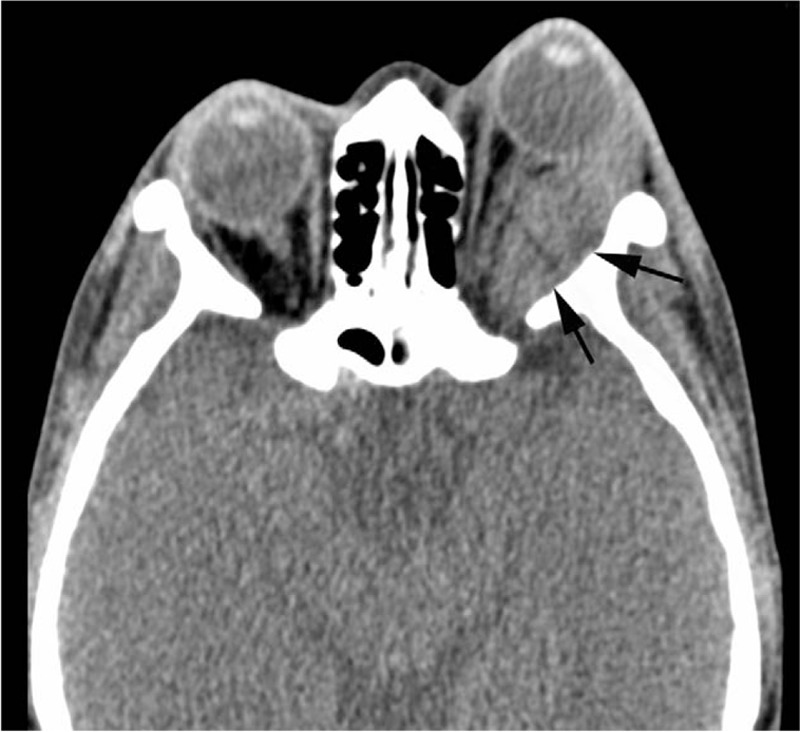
CT scan revealed a larger mass out of the left eye muscular cone space (the mass is indicated by arrows, 4.4 × 1.7 cm). CT = computed tomography.
